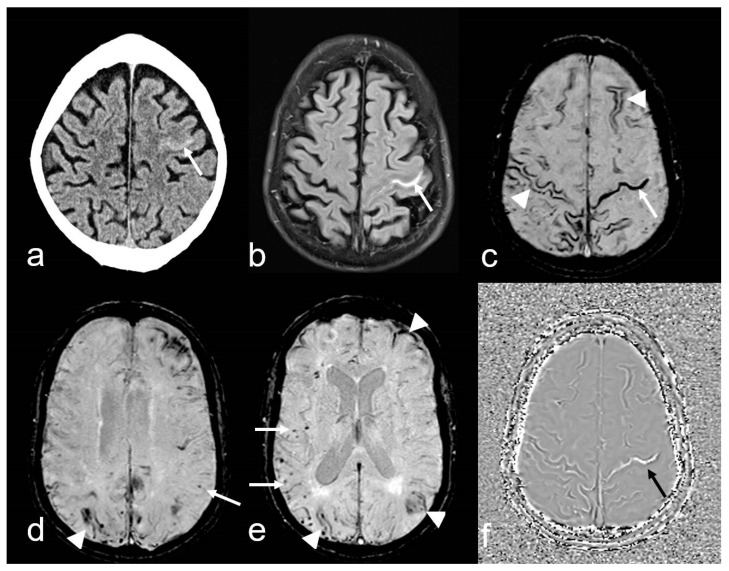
A 74-year-old man with progressive cognitive impairment suffering from temporary sensory–motoric deficits right. Cortical subarachnoid hemorrhage (cSAH) in the central sulcus ((a), CT: arrow; Siemens Somatom Emotion). MRI with sulcal hyperintense signal changes on fluid attenuated inversion recovery (FLAIR) images ((b), arrow), sulcal signal loss on susceptibility-weighted imaging (SWI, (c): arrow), additional multifocal cortical superficial siderosis (cSS) bilateral ((c–e), arrowhead); note the characteristic bilinear track-line appearance of cSS in the chronic stage ((c), arrowhead); (d,e): multiple cortical/subcortical microbleeds (MB, arrow); (f): SWI-phase image demonstrating paramagnetic effects in the central sulcus due to blood degeneration products (arrow); MRI 1.5 T Siemens AREA.
